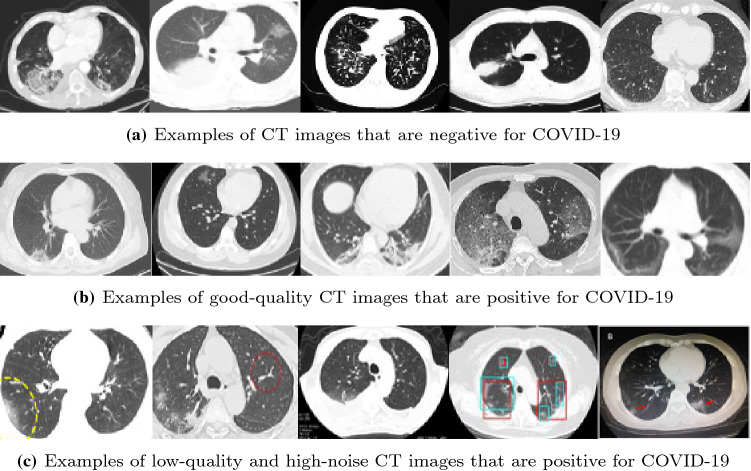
Examples of CT images from COVID-CT data set
